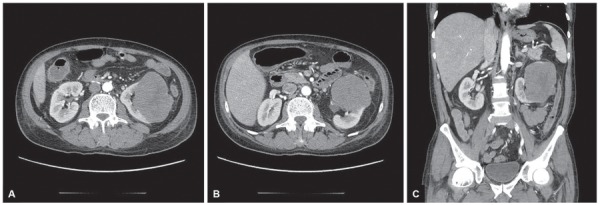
Computed tomography scans revealed a giant left kidney tumor
